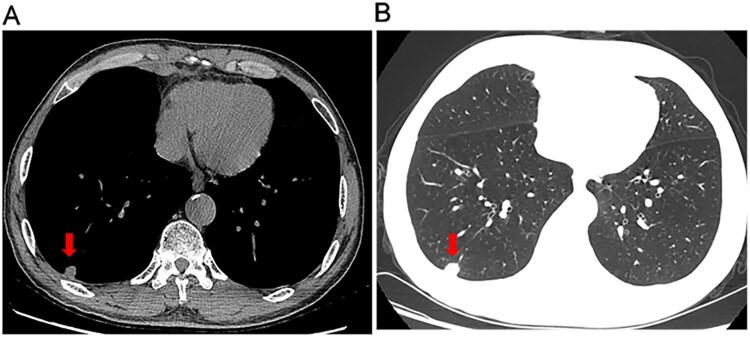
Chest computed tomography (CT) scans. CT images of the lung window (A) and soft tissue window (B) revealed a 1.4 × 1.3 × 1.1 cm solitary solid nodule in the left lower lobe (arrowhead), mild ground-glass opacity and multiple small nodules in both lungs.
